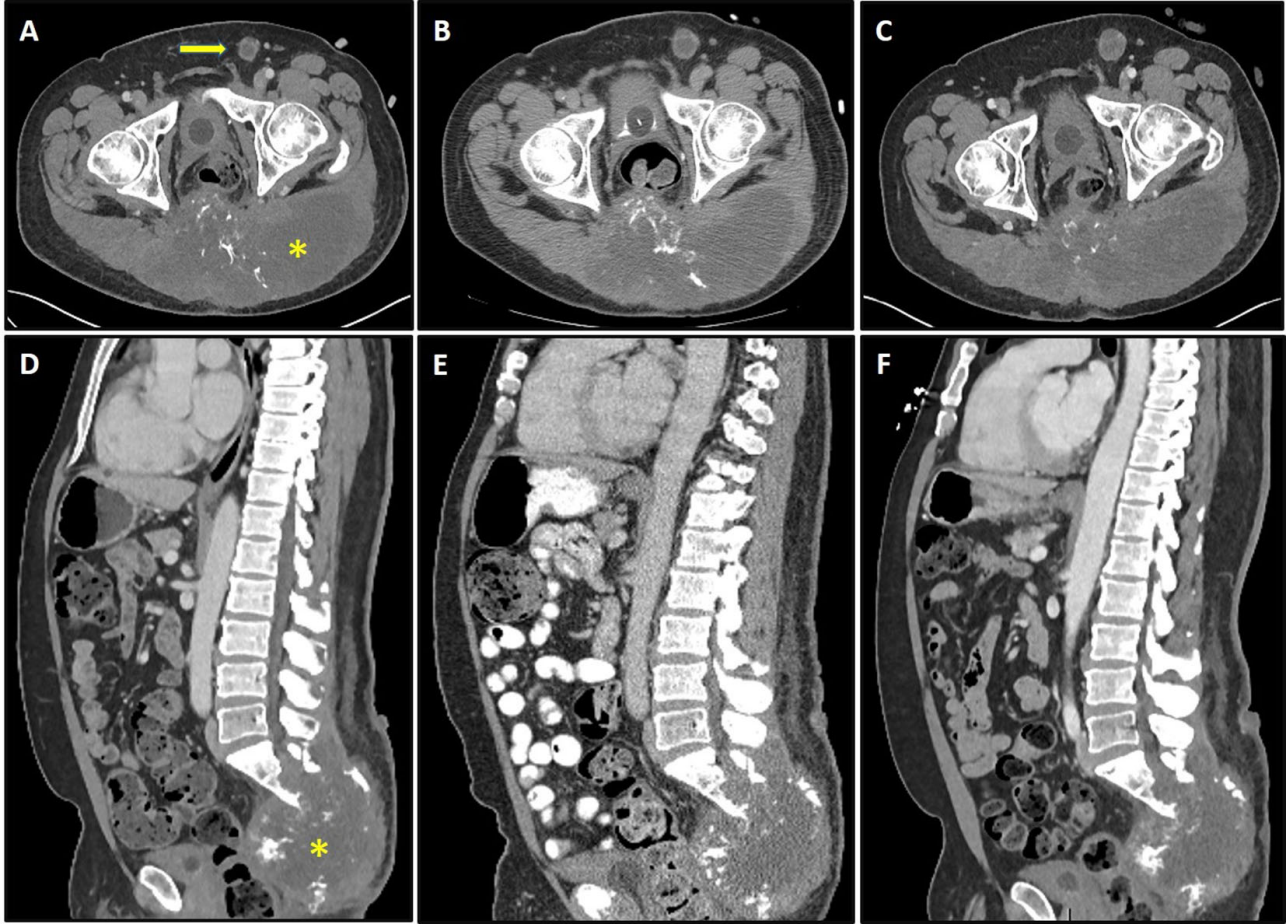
Axial and sagittal sections of CT scans depicting sacral chordoma (asterisk) with left inguinal lymphadenopathy (arrow) prior to brachyury vaccine (A, D), 4 weeks after the first dose of vaccine (B, E), and 16 weeks after the first dose of vaccine (C, F). Imaging demonstrates stable disease by response evaluation criteria in solid tumors (RECIST) criteria, with 1.54 and 3.38% changes in baseline at 4 weeks (B, E) and 16 weeks (C, F), respectively.
